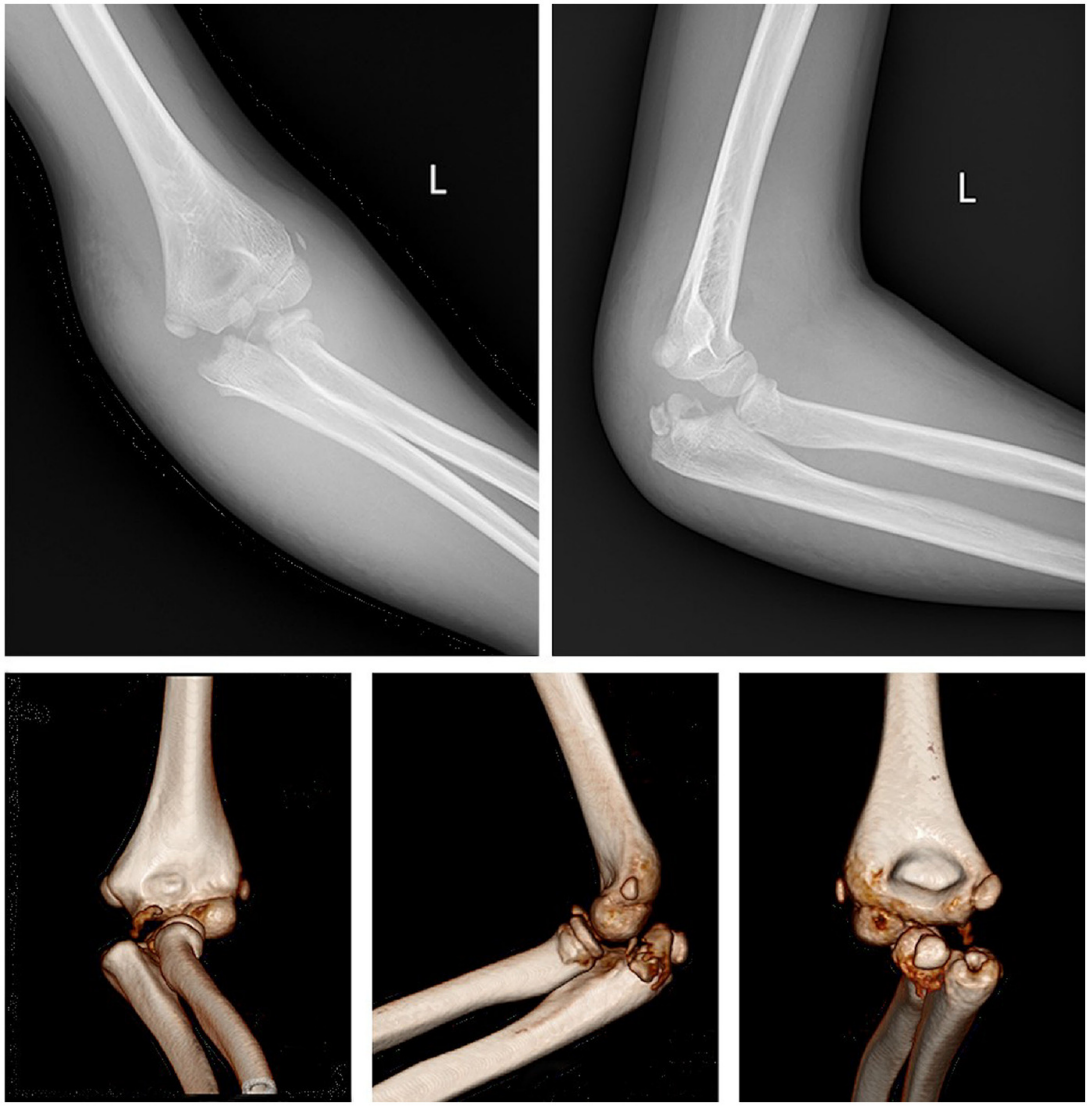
X-rays and CT scans showed fractures in the coronoid process, olecranon of the ulna, and the radial neck, along with dislocation of radiocapitellar joint and posterior medial displacement of proximal radial-ulnar joint.
